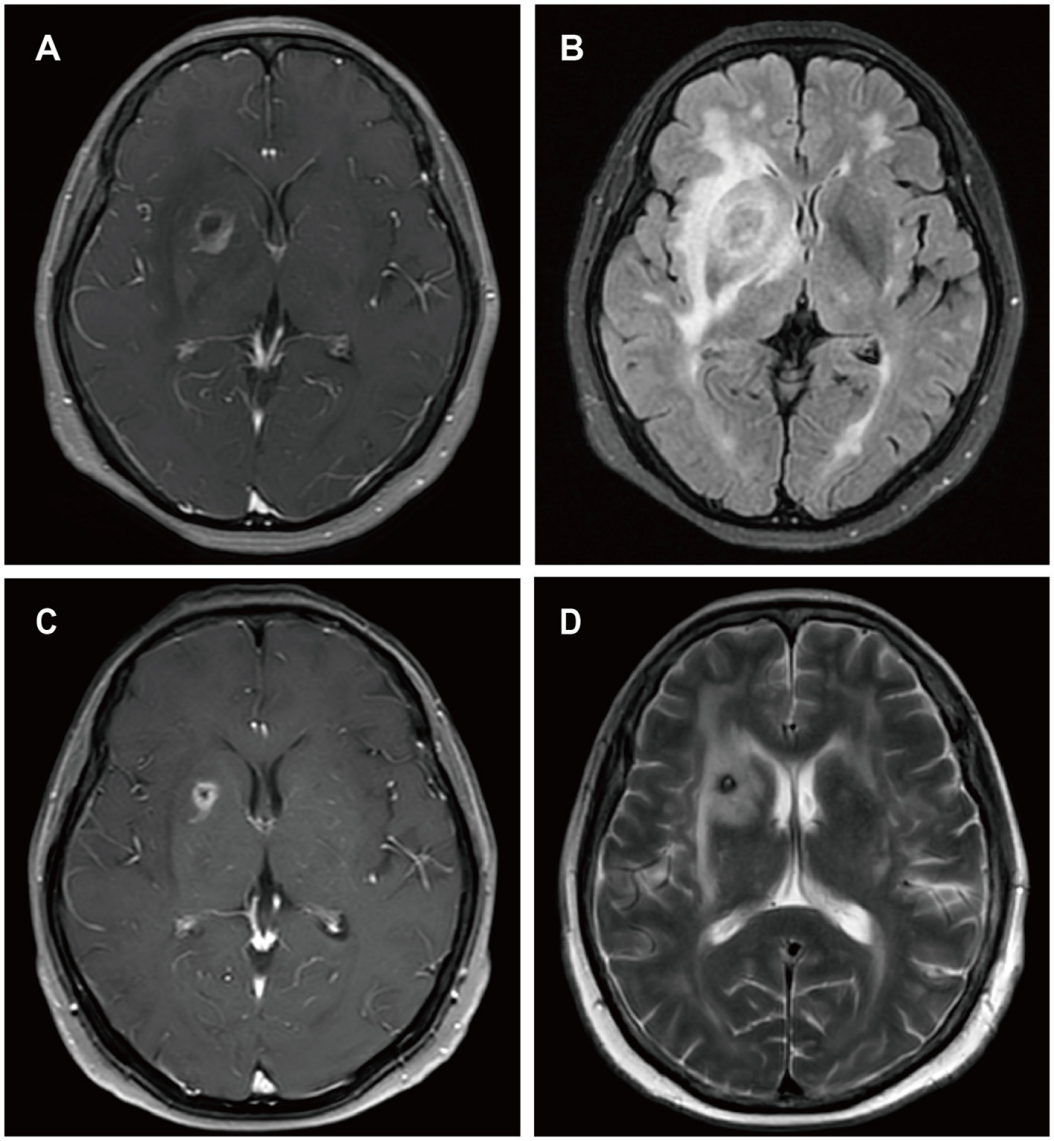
MRI findings of patient 4. Preoperative enhanced MRI prior to brain biopsy showed a ring-enhancing lesion in the right basal ganglia area on T1-weighted images (A), with extensive edema surrounding the lesion in the right basal ganglia area on T2-FLAIR images (B). On follow-up enhanced MRI images obtained 3 months after the diagnosis of PCNS PTLD, the ring-enhancing lesion in the right basal ganglia area appeared reduced on T1-weighted images (C), and the edema surrounding the lesion in the right basal ganglia area decreased on T2-weighted images (D).
